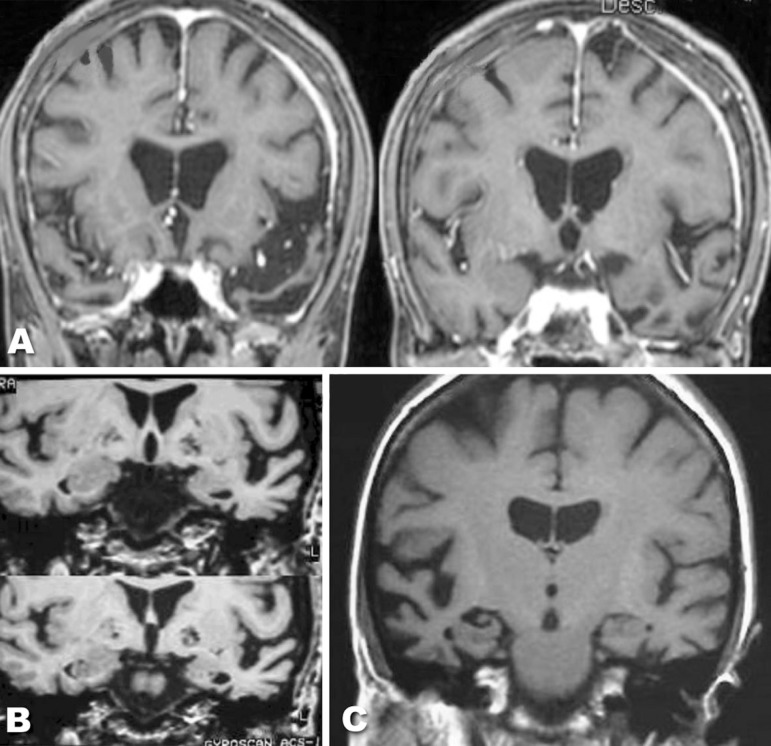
Examples of structural imaging (MRI) of three cases with semantic
